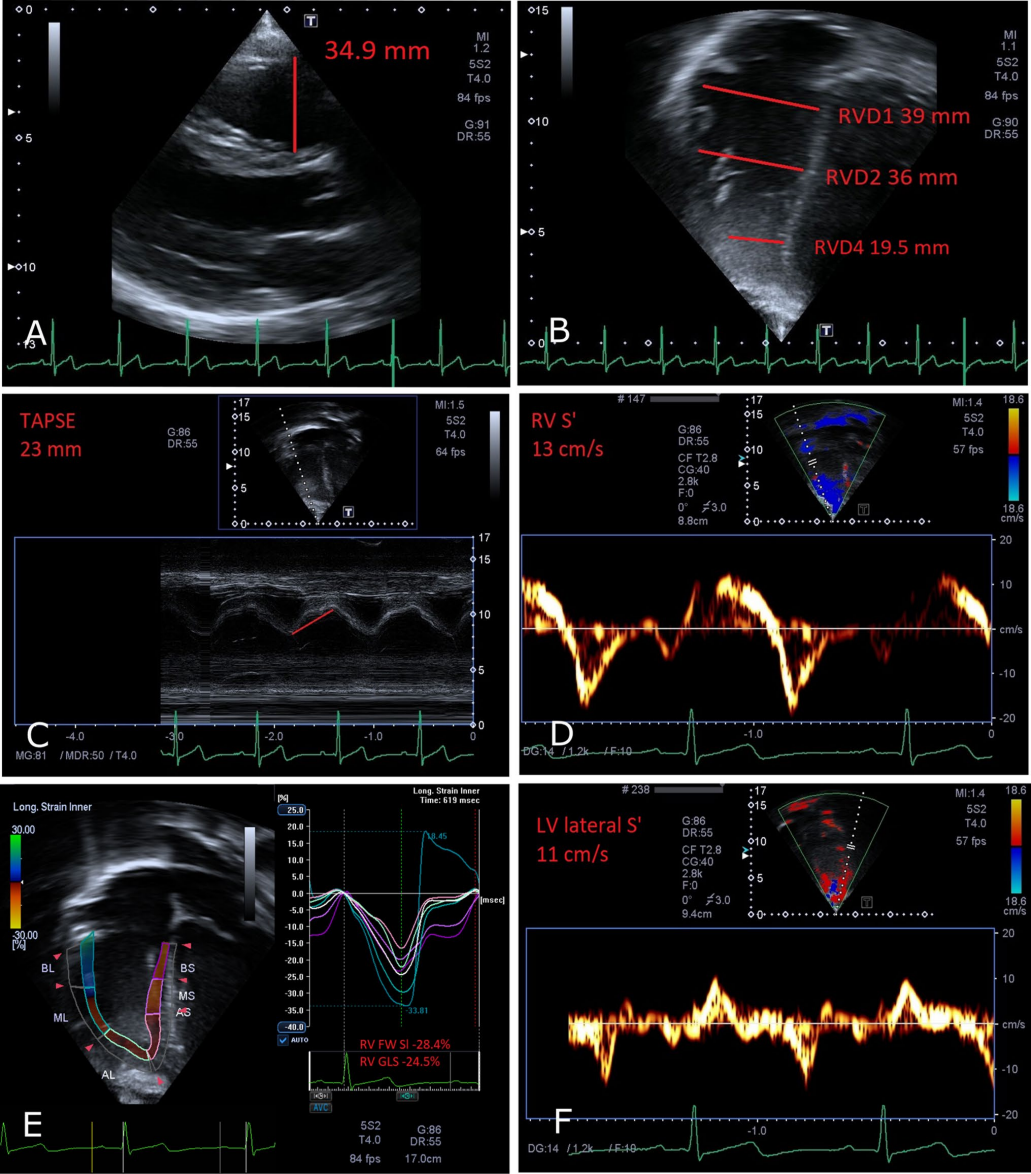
Echocardiographic biventricular size and systolic function measurements in Athlete 1. A PLAX view showing RVOT end-diastolic diameter. B A4C view showing RV basal (RVD_1_), mid (RVD_2_), and apical (RVD_4_) end-diastolic diameters. C M-mode view showing TAPSE. D Tissue Doppler imaging showing the peak systolic tricuspid annular velocity (RV S’). E Speckle-tracking echocardiography showing RV segmental strain curves, free-wall RV strain (RV Sl), and global RV strain (RV GLS). F Tissue Doppler imaging showing the peak systolic mitral lateral annular velocity (LV lateral S’)
